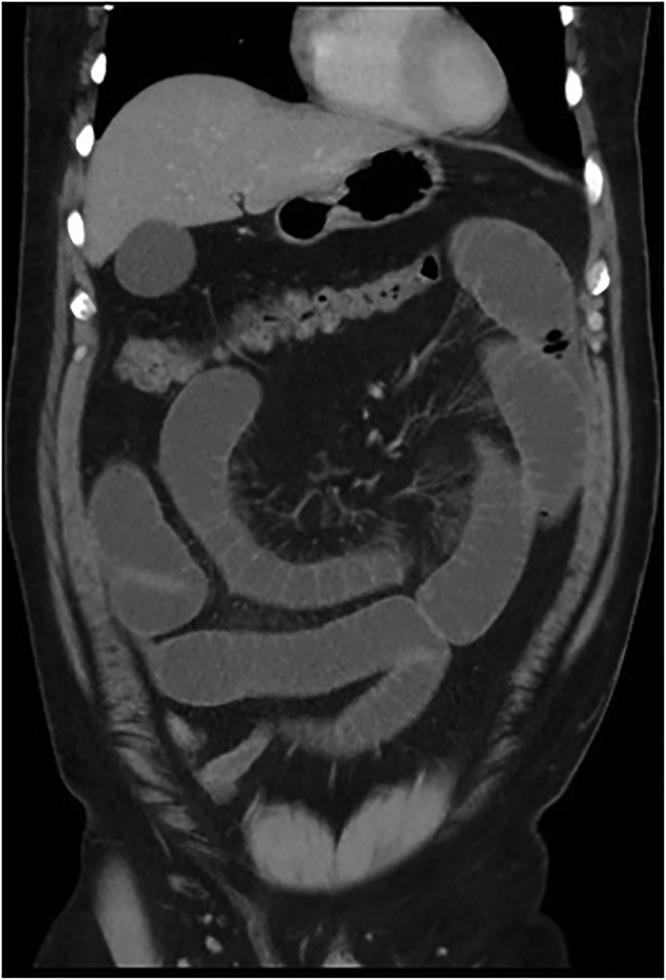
Coronal view of the CT abdomen and pelvis showing multiple dilated small bowel loops consistent with SBO.
